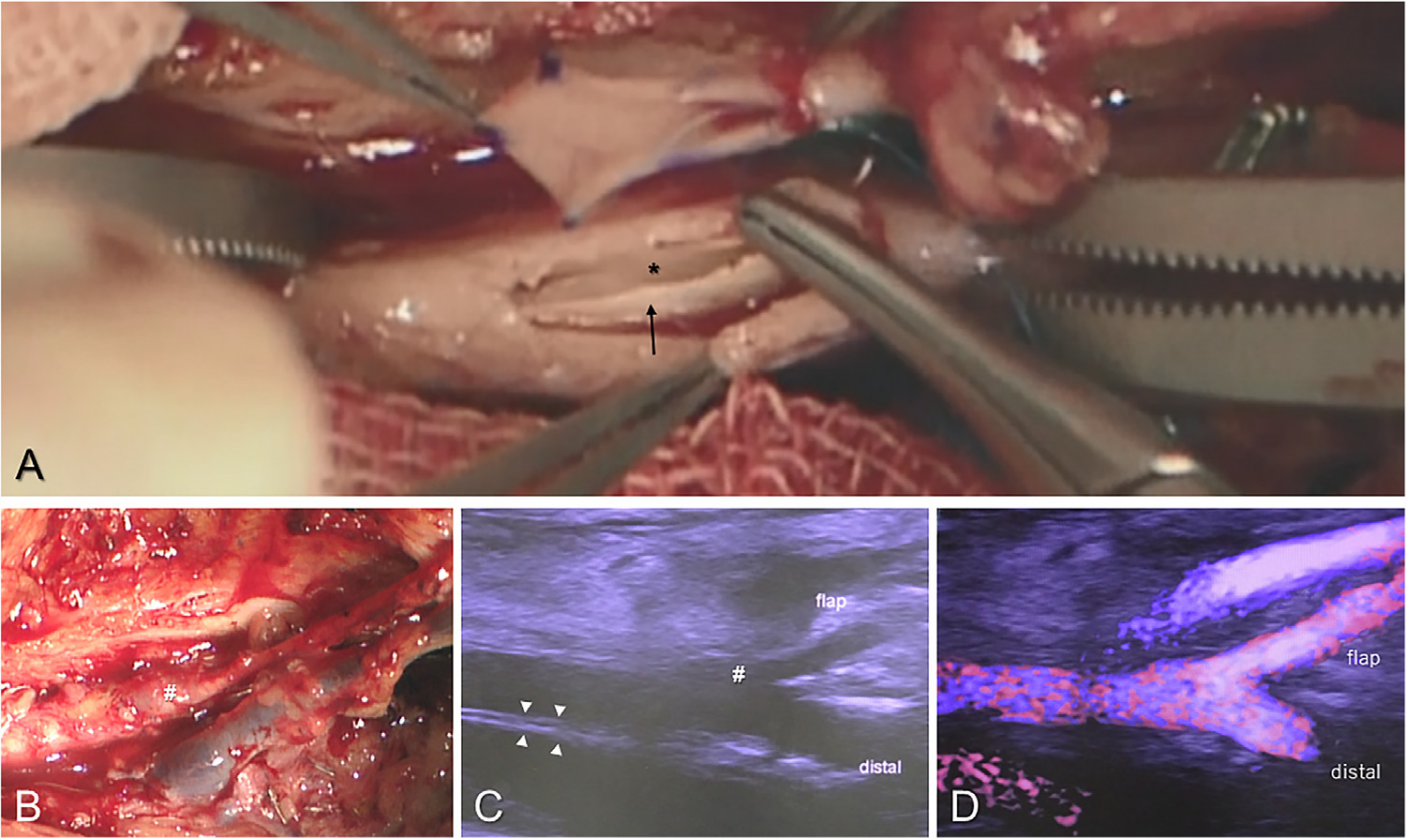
Arterial anastomosis to the atherosclerotic SFA (case 2). A: Clear visualisation of the denuded intima (black arrow) and true lumen of the vessel (asterisk) through a large window. By directing the needle away from the vessel lumen, precise suturing can be achieved without stripping the vessel's intima. B: Post-anastomosis appearance showing the bulging anastomosis site (hashmark) over the thick SFA. C: Ultrasound image at 3 weeks after flap surgery revealing a well-expanded window on the thick arterial wall (arrowhead). D: Colour Doppler showing no turbulence at the anastomosis site, ensuring good blood flow to the flap and recipient periphery.
